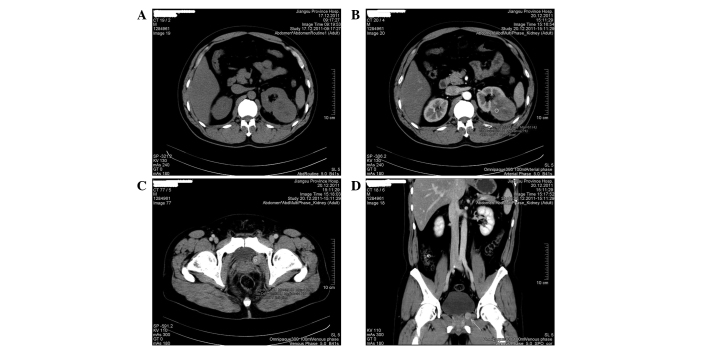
Abdominal computed tomography scan prior to the initial surgery showed (A) a roughly circular and well-demarcated mass with (B) clear enhancement effect (5.4×4.5 cm^2^ at the maximum diameter) in the lower pole of the left kidney and an additional oval-shaped mass (1.5×1.0 cm^2^ at maximum diameter) on the left side of the prostate in the (C) horizontal plane and (D) coronal plane..
